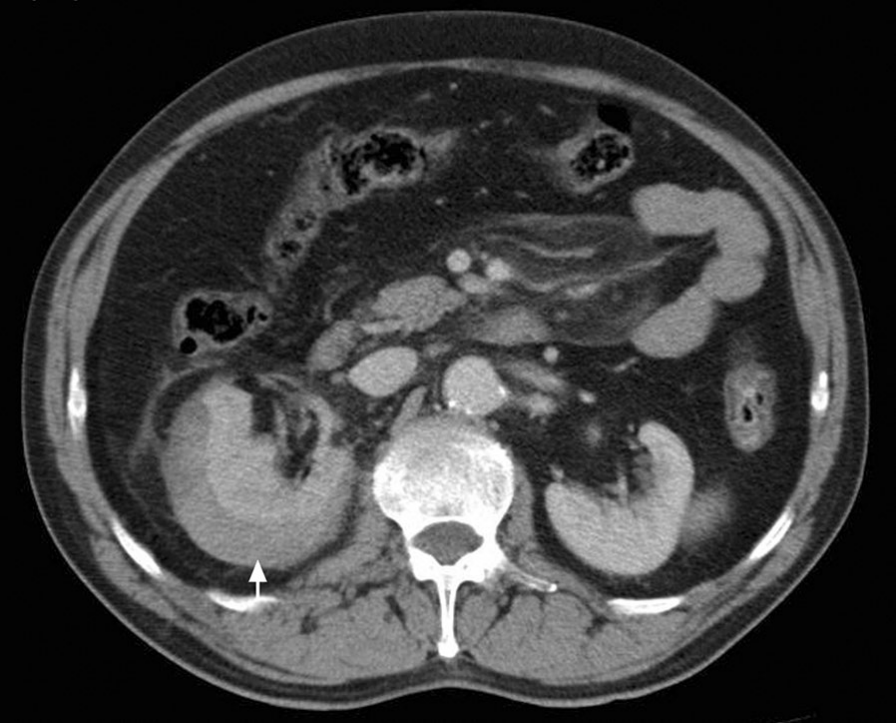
CT scan of the abdomen. Sixty-one-year-old male 24 h after ESLW presenting with acute right lumbar pain. Contrast CT showing a subcapsular collection in the right kidney (arrow), compatible with subcapsular renal hematoma, without other renal or abdominal complications
